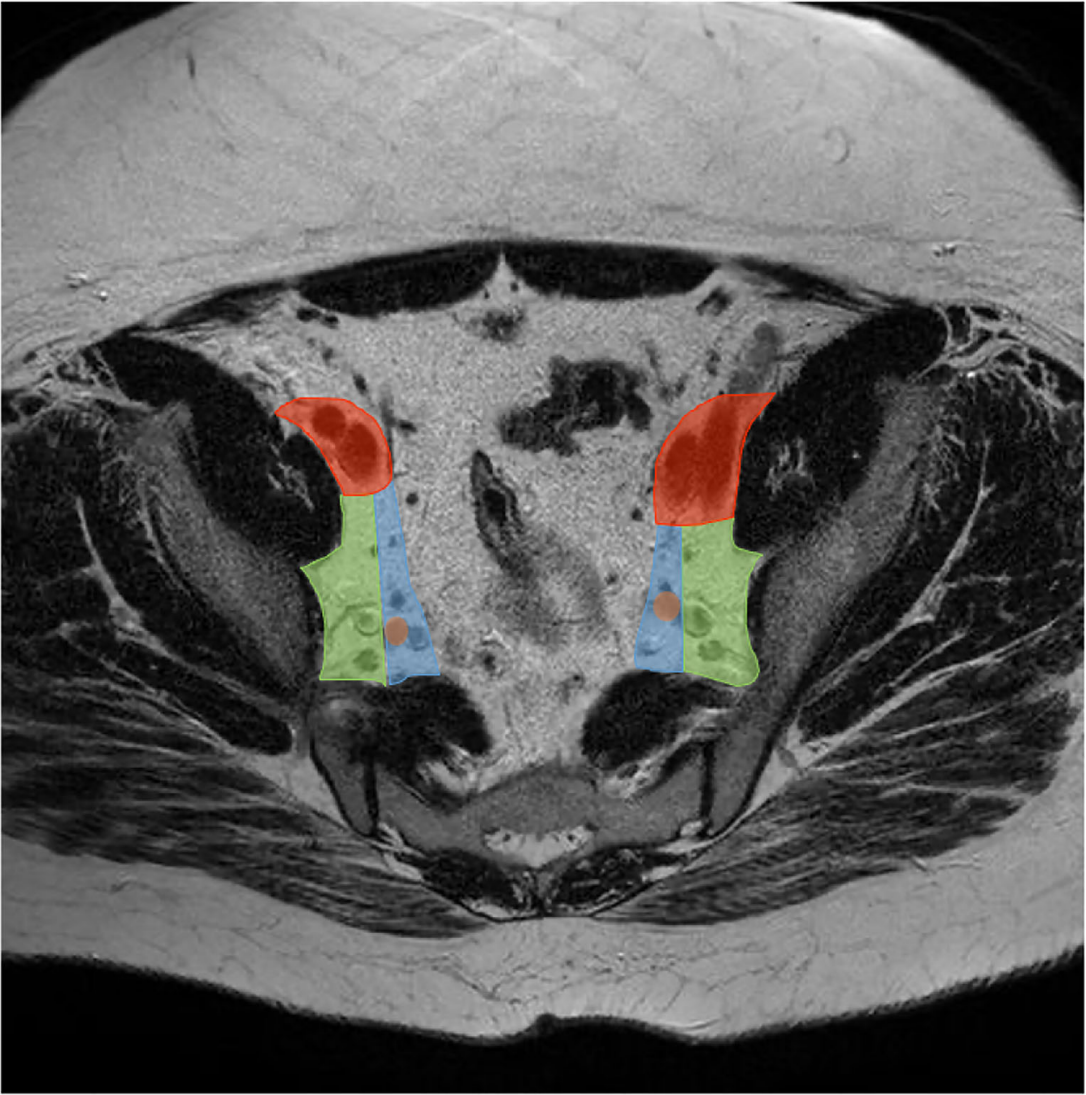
Axial view of T2-MRI depicting the lateral compartments. Red—external iliac compartment, green - obturator compartment, blue—internal iliac compartment. Brown spot: primary trunk of the internal iliac artery. The internal iliac compartment is defined as medial to the lateral border of the primary trunk of the internal iliac artery. The obturator compartment is the tissue located laterally of the primary trunk of the internal iliac artery and all tissue still present after the internal iliac artery exits the pelvis
